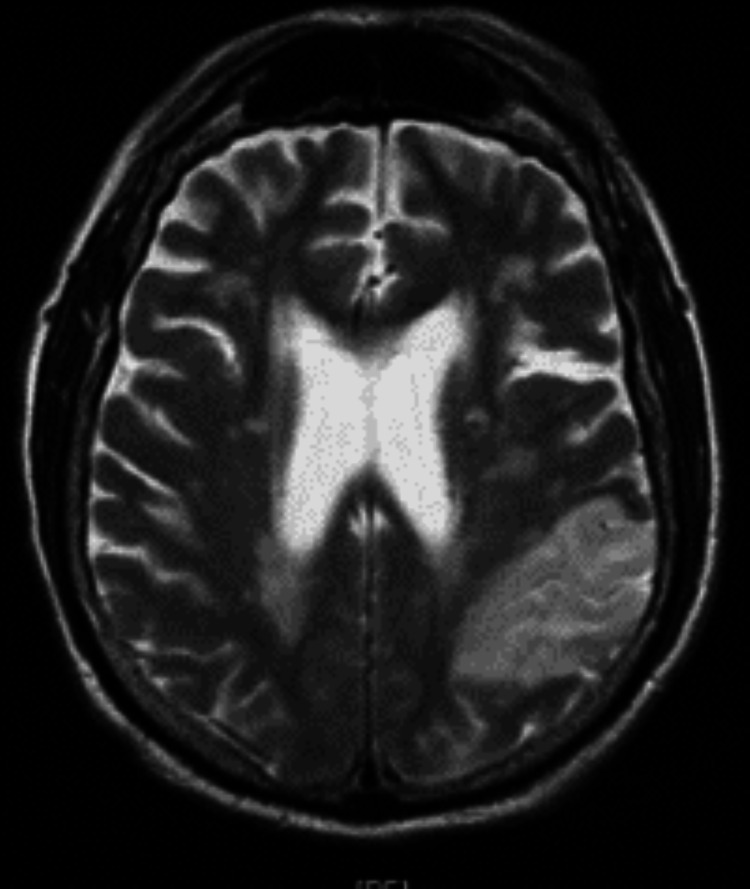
T2-weighted MRI showing T2 signal abnormality
